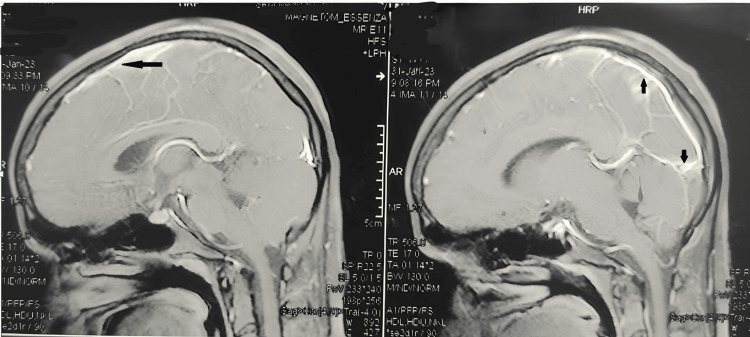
Contrast-enhanced MRI brain images Leptomeningeal enhancement is seen in the images (black arrows).
